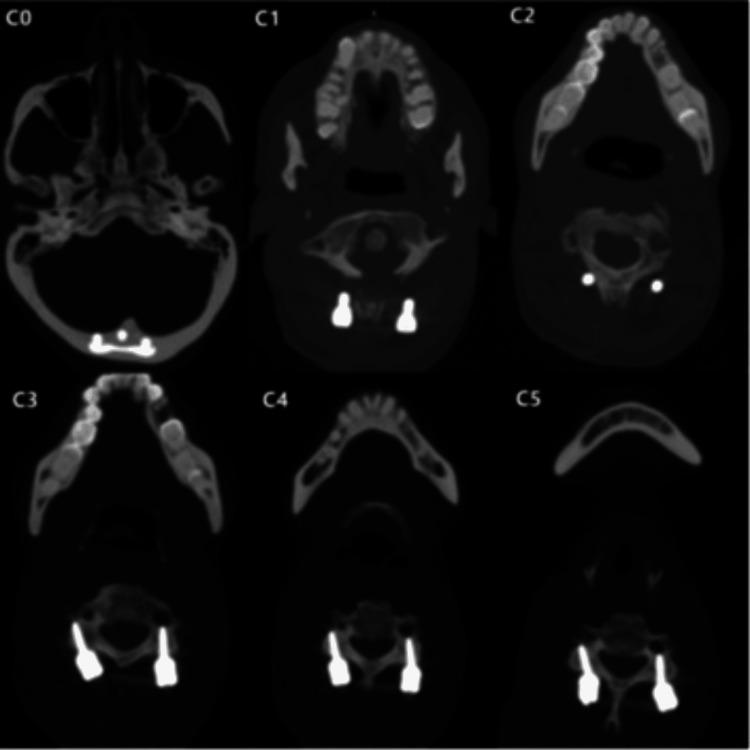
Non-contrast CT of the skull and cervical spine with bone window verifying the entry position of the fixation screws using the Magerl technique.
